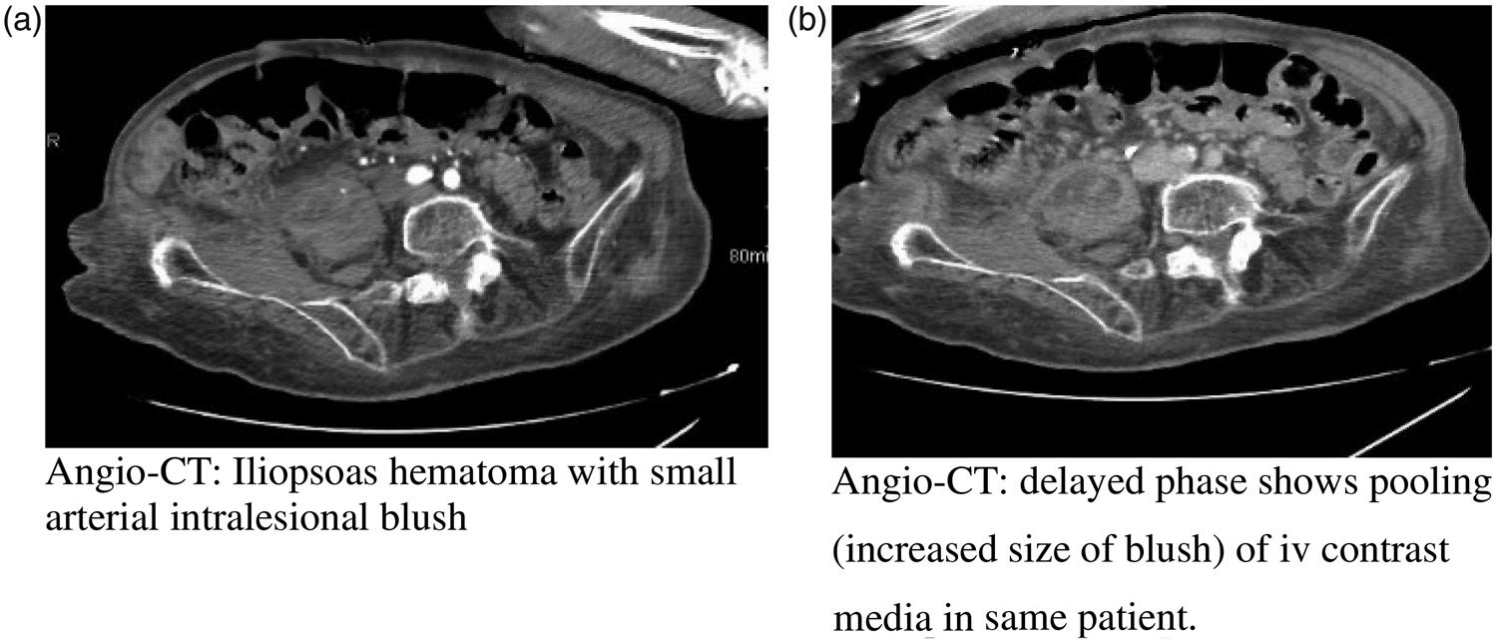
(a) Arterial phase CT of right haematoma and (b) delayed phase CT of right haematoma.
